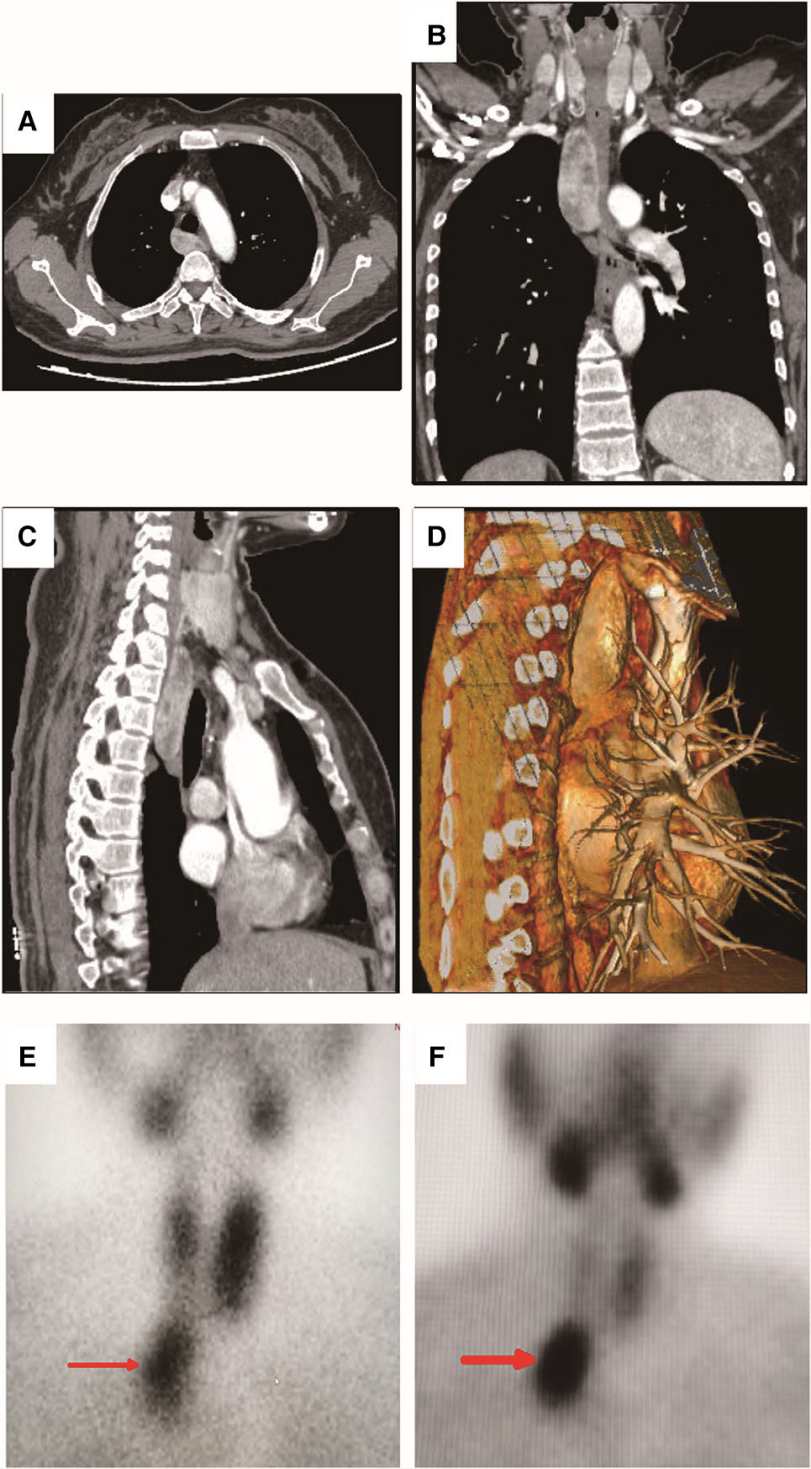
Computed tomography (CT) scan and 99^m^-Technetium sestamibi scanning of the patient. (A) Transverse view of the CT scanning showed a mixed density mass with uneven enhancement in the posterior mediastinum. The maximum cross-section size was about 3.3×1.9 cm.; (B) Coronal view of the CT scanning; (C) Sagittal view of the CT scanning; (D). Three-dimensional reconstruction in sagittal view of the CT scanning; (E) The 15th minute static imaging: the left thyroid lobe was more prominent, whereas the uptake of imaging agent in the right thyroid lobe was decreased; In addition, localized abnormal uptake of imaging agent under the right thyroid lobe was found (as shown by the red arrow). (F) The two-hour delayed imaging: the uptake of thyroid imaging was lower than that before, the abnormal imaging agent concentration under the right thyroid lobe was clearly displayed (as shown by the red arrow).
